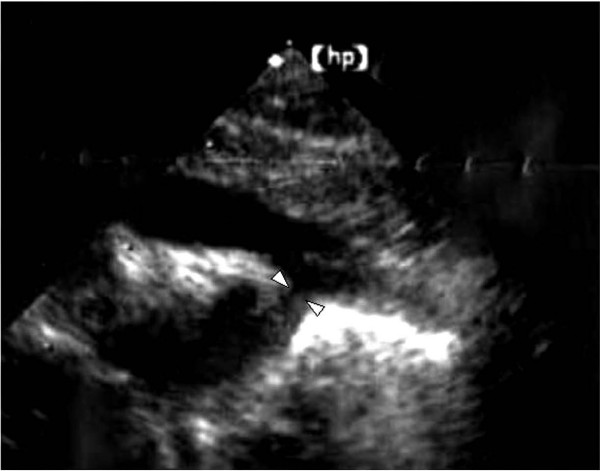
Echocardiography demonstrated a 1.5 mm diameter preductal coarctation of the aorta (CoA) (arrows).
